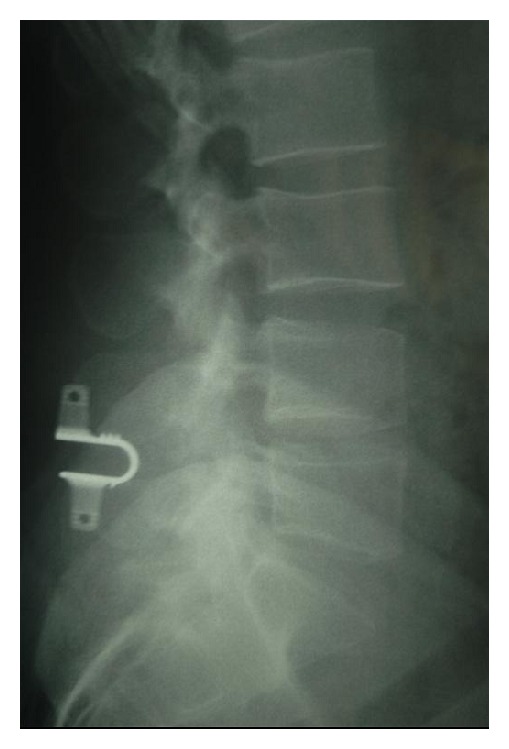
Lateral X-ray image of a Coflex implant.
